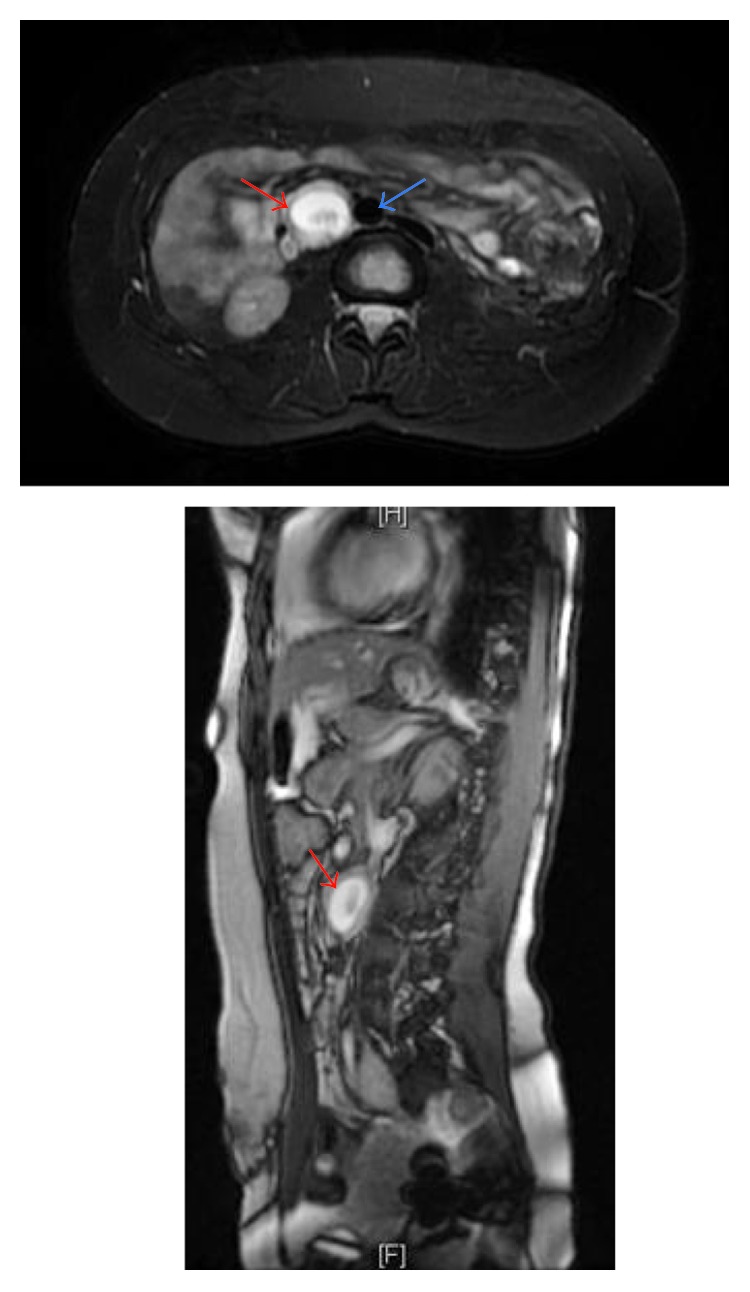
MRI scan in T2 mode showing the gestational sac (red arrow) in the left para-aortic (blue arrow) region.
